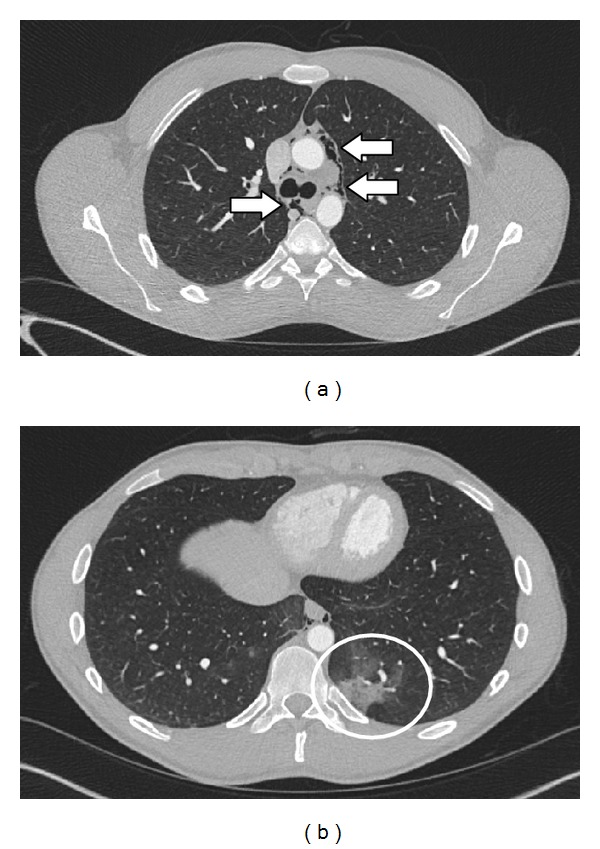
Chest computed tomography showing air outlining mediastinal structures (arrows) and a small pulmonary contusion on the left side (circle).
